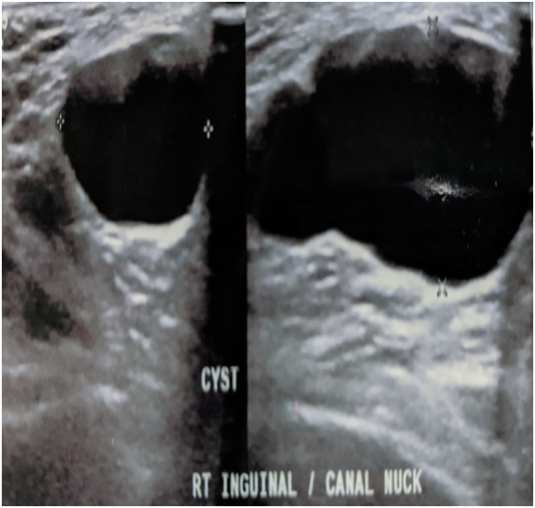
Ultrasound picture showing cyst in the canal of Nuck with septae.
